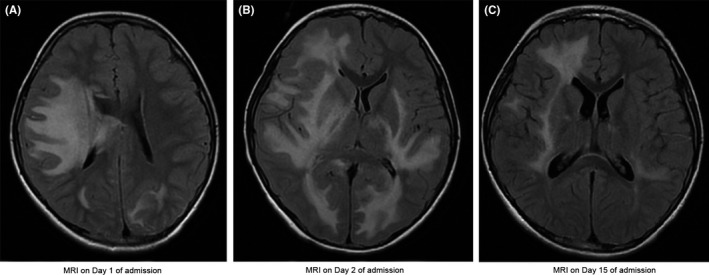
A, MRI (on Day 1 of admission) showing patchy enhancement of cerebral white matter associated with a mild right to left midline shift. B, MRI (on Day 2 of admission) showing a marked progression of diffuse cerebral edema of bilateral white matter, right more than left, with worsening of a midline shift. C, MRI (on Day 15 of admission) showing significant improvement in cerebral edema (less hyperintense white matter area) with a near complete resolution of a midline shift
